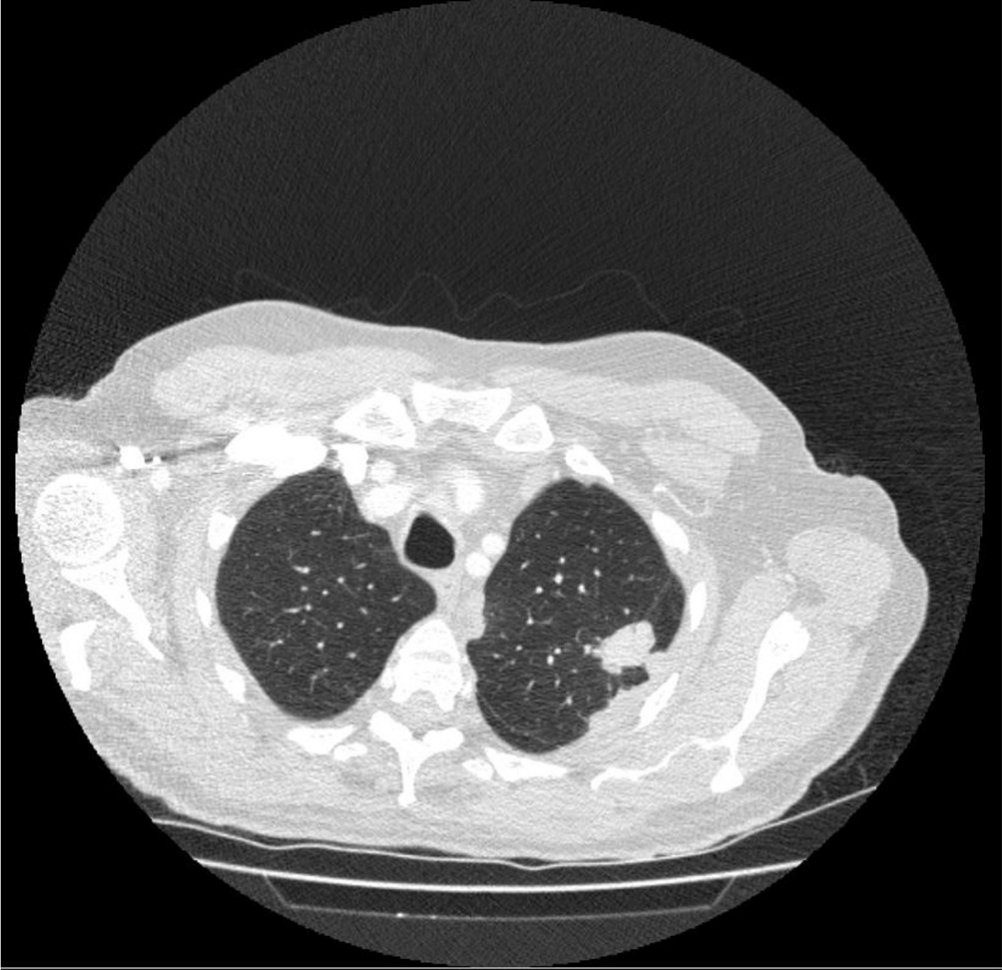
T*_1 _weighted axial MRI section showing invasion of vertebral body from the mass causing compression of the spinal cord at T5 level.
